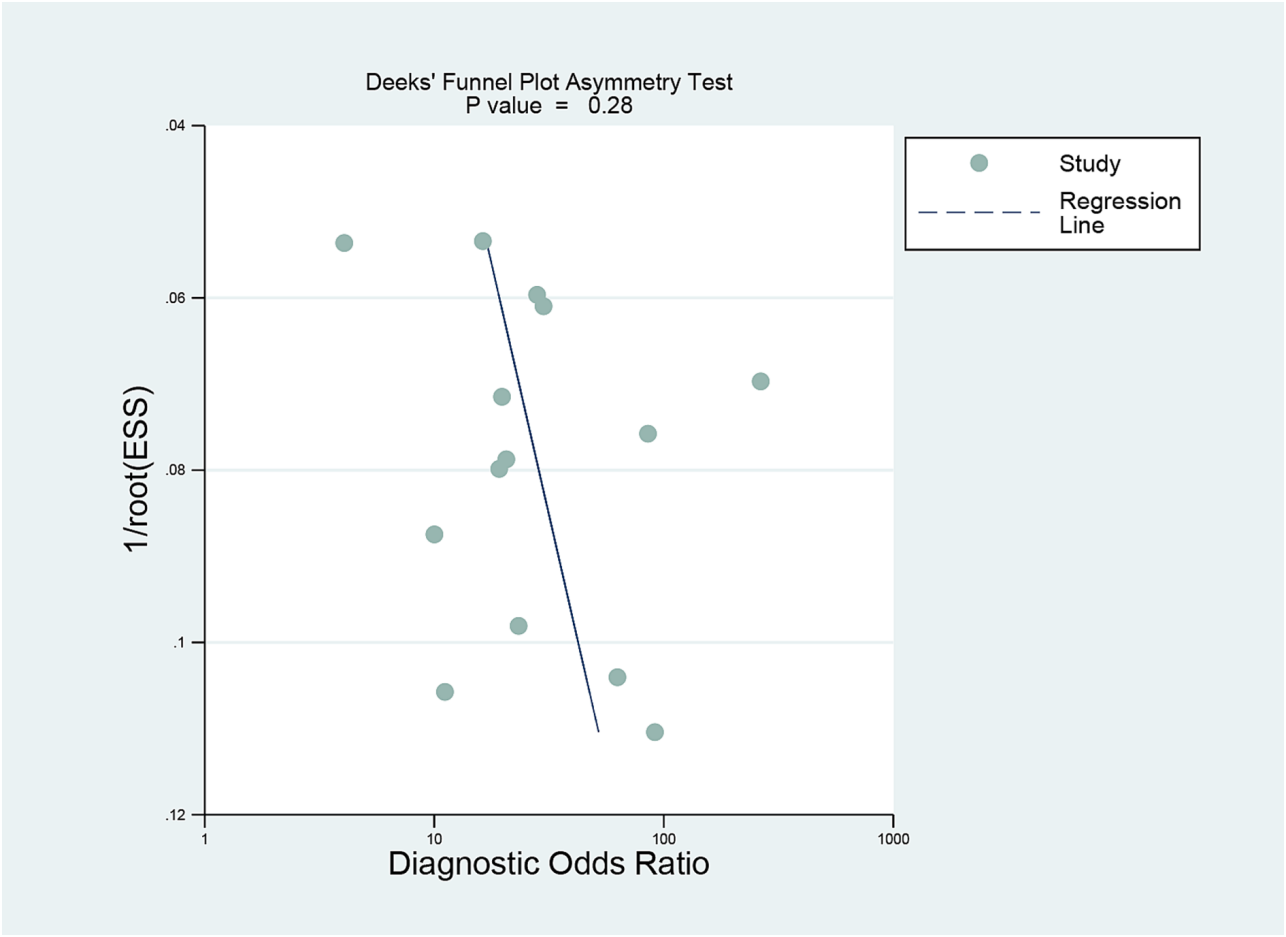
Deek’s funnel plot was used to evaluate the publication bias of DECT. *P* < 0.05 was considered significant. DECT, dual-energy computed tomography.
